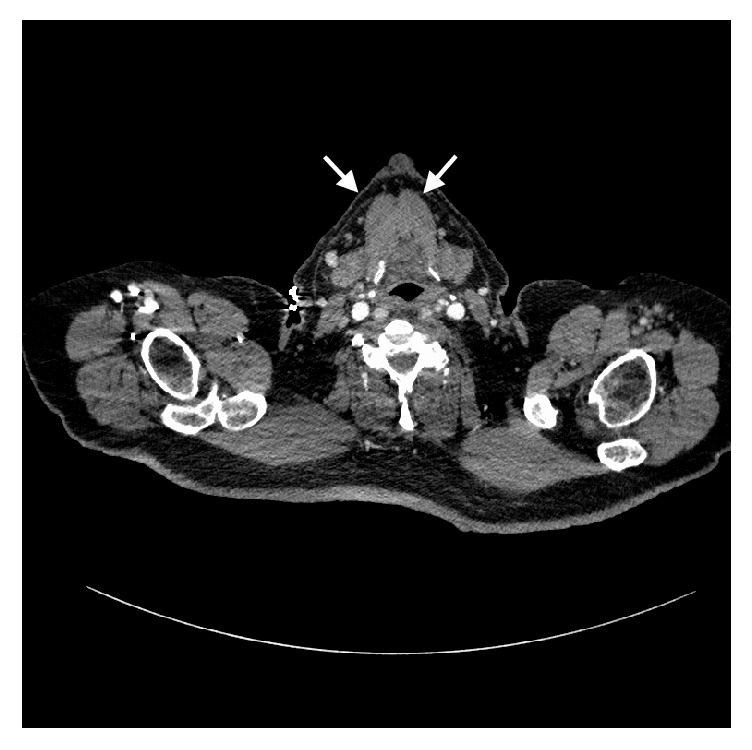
CT scan of neck with contrast showing mildly enlarged and heterogeneous thyroid glands (white arrows) (right>left) with surrounding hazy changes.
